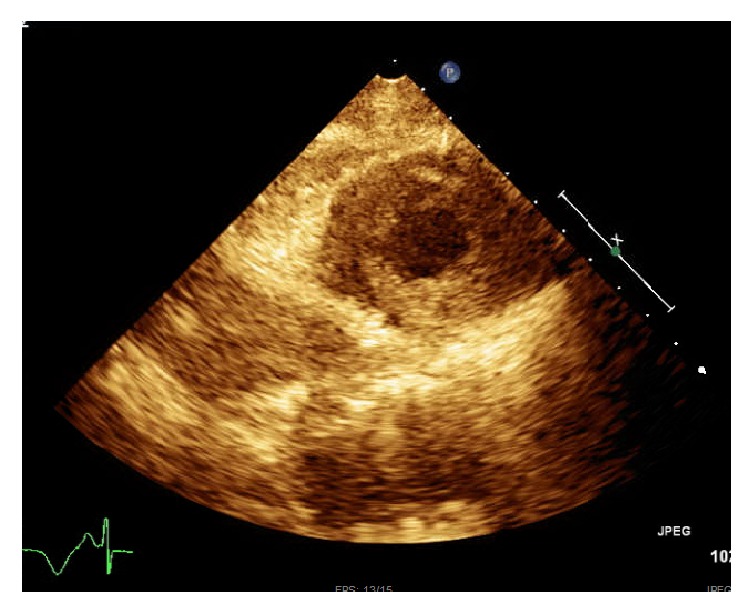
Echocardiogram showing apical hypokinesia.
